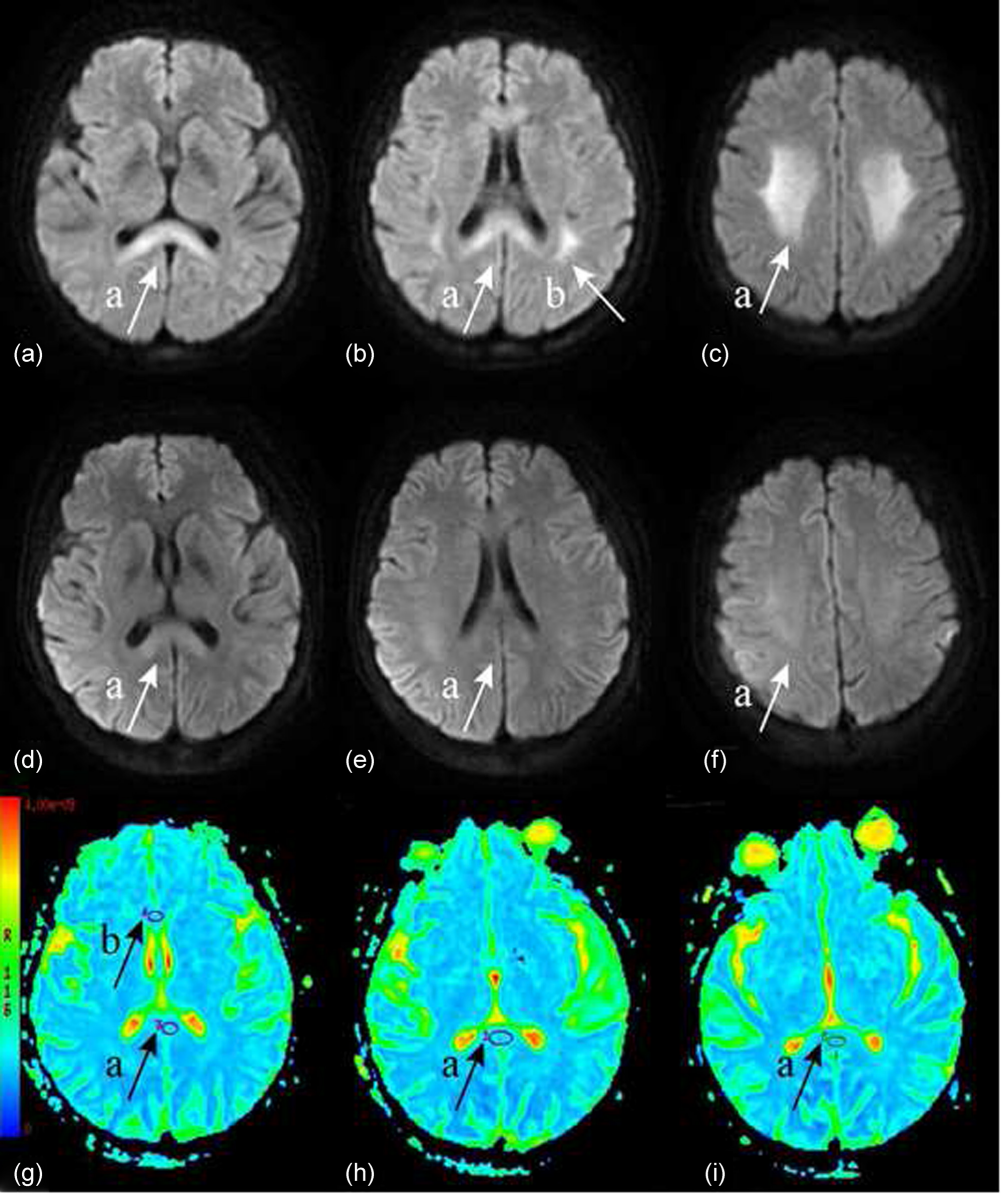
Magnetic resonance images. (a-a/b-a) DWI of the corpus callosum. The signal is limited, showing a “boomerang sign” on day 9; (b-b) DWI showing high signal shadows in the lateral ventricle on day 9; (c-a) DWI showing limited signal in the bilateral semioval center on day 9; (d-a/e-a) DWI showing that the high signal in the corpus callosum has basically disappeared. (f-a) The bilateral semi-oval center lesions are significantly reduced. (g-a/h-a/i-a) The region of interest (ROI) in the splenium of the corpus callosum. (g-b) The genu of the corpus callosum as a control.
